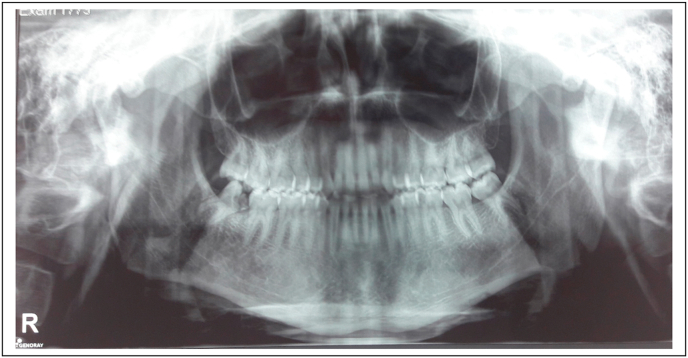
Preoperative radiograph showing the impacted mandibular right third molar.
